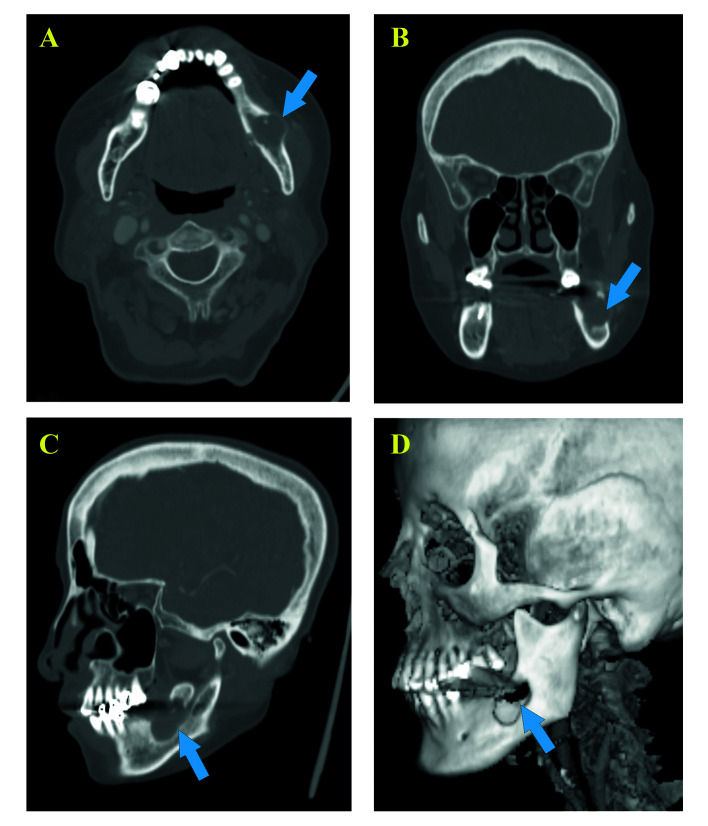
Spiral computed tomography (SCT) findings of PIOSCC ex OKC in the left body of the mandible. (A) Axial plane view showing local lysis of lingual cortex and trabeculae, lytic expansion of buccal cortex. (B, C) Coronal and Sagittal plane views showing local osteolysis and involvement of mandibular canal wall. (D) Three-dimensional image showing an osteolytic lesion with relatively irregular boundaries in the left molar area of the mandible.
